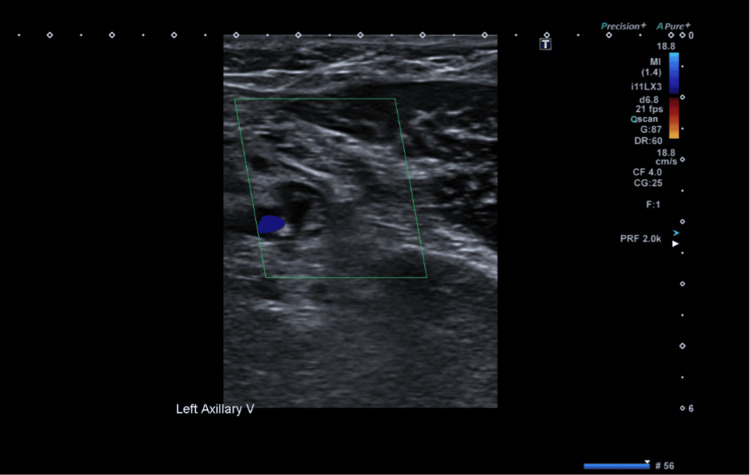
US shows no colour Doppler flow in the axillary vein (green box) US: ultrasound
